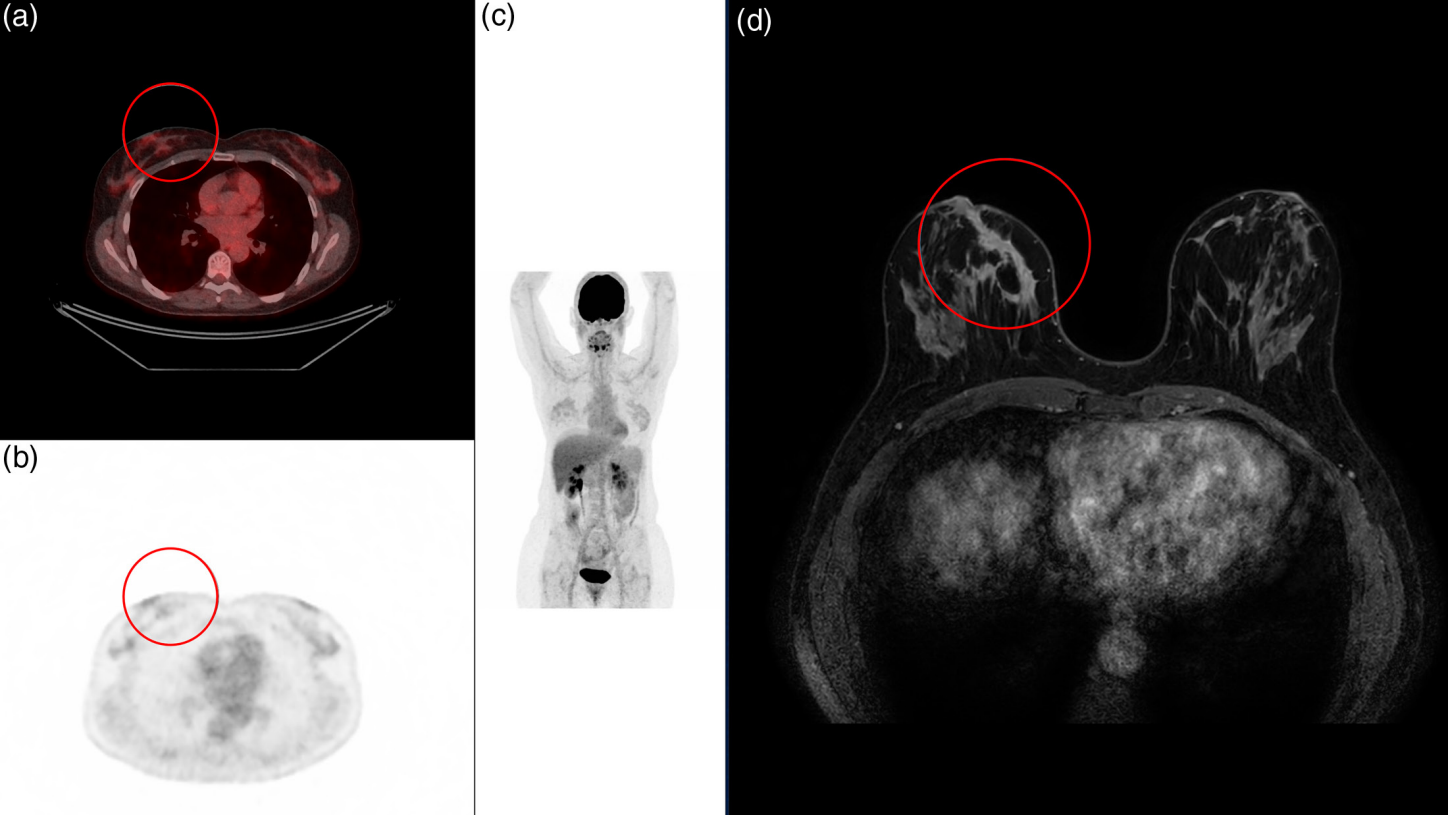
Baseline [^18^F]FDG PET/CT exam of a 39-year-old patient with right estrogen receptor–positive lobular breast cancer with a low metabolic uptake (SUV_max_ primary tumour = 1.53). The breast lesion was not visible on [^18^F]FDG PET/CT (a, axial fusion image; b, axial PET image; c, maximum intensity projection image), but was detected on MRI (d, axial contrast-enhanced T1-weighted MRI exam). CT, computed tomography; [^18^F]FDG, [^18^F] fluorodeoxyglucose; SUV_max_, maximum standardised uptake value.
